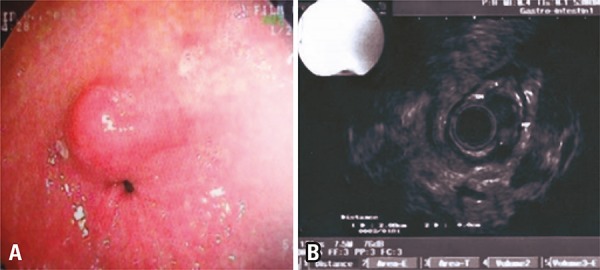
(A) Endoscopy showing a bulge in gastric antrum adjacent to the pylorus. (B) Endoscopic ultrasound revealed the lesion as compatible with a gastrointestinal stromal tumor, but abdominal computed tomography was recommended
